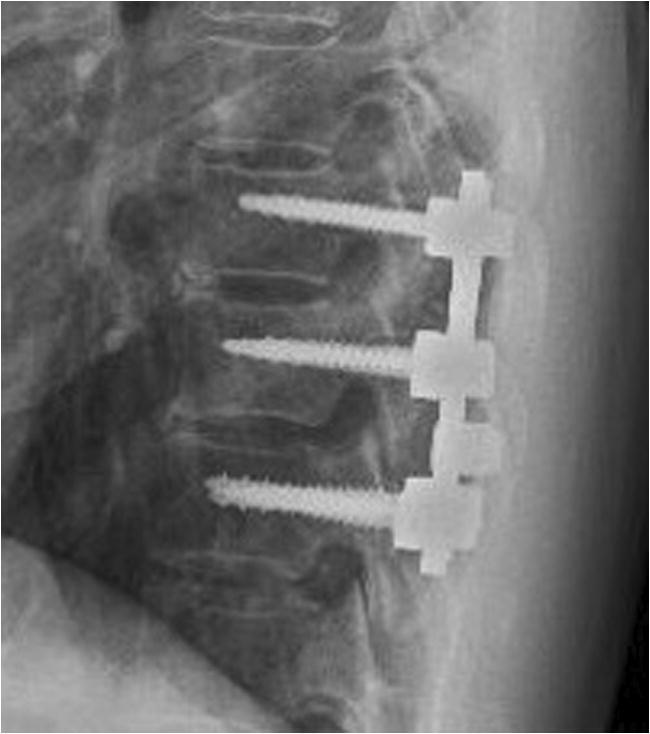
Lateral view of the postoperative plain radiograph. Vertebral decompression and posterolateral fixation of Th9–11 were performed
